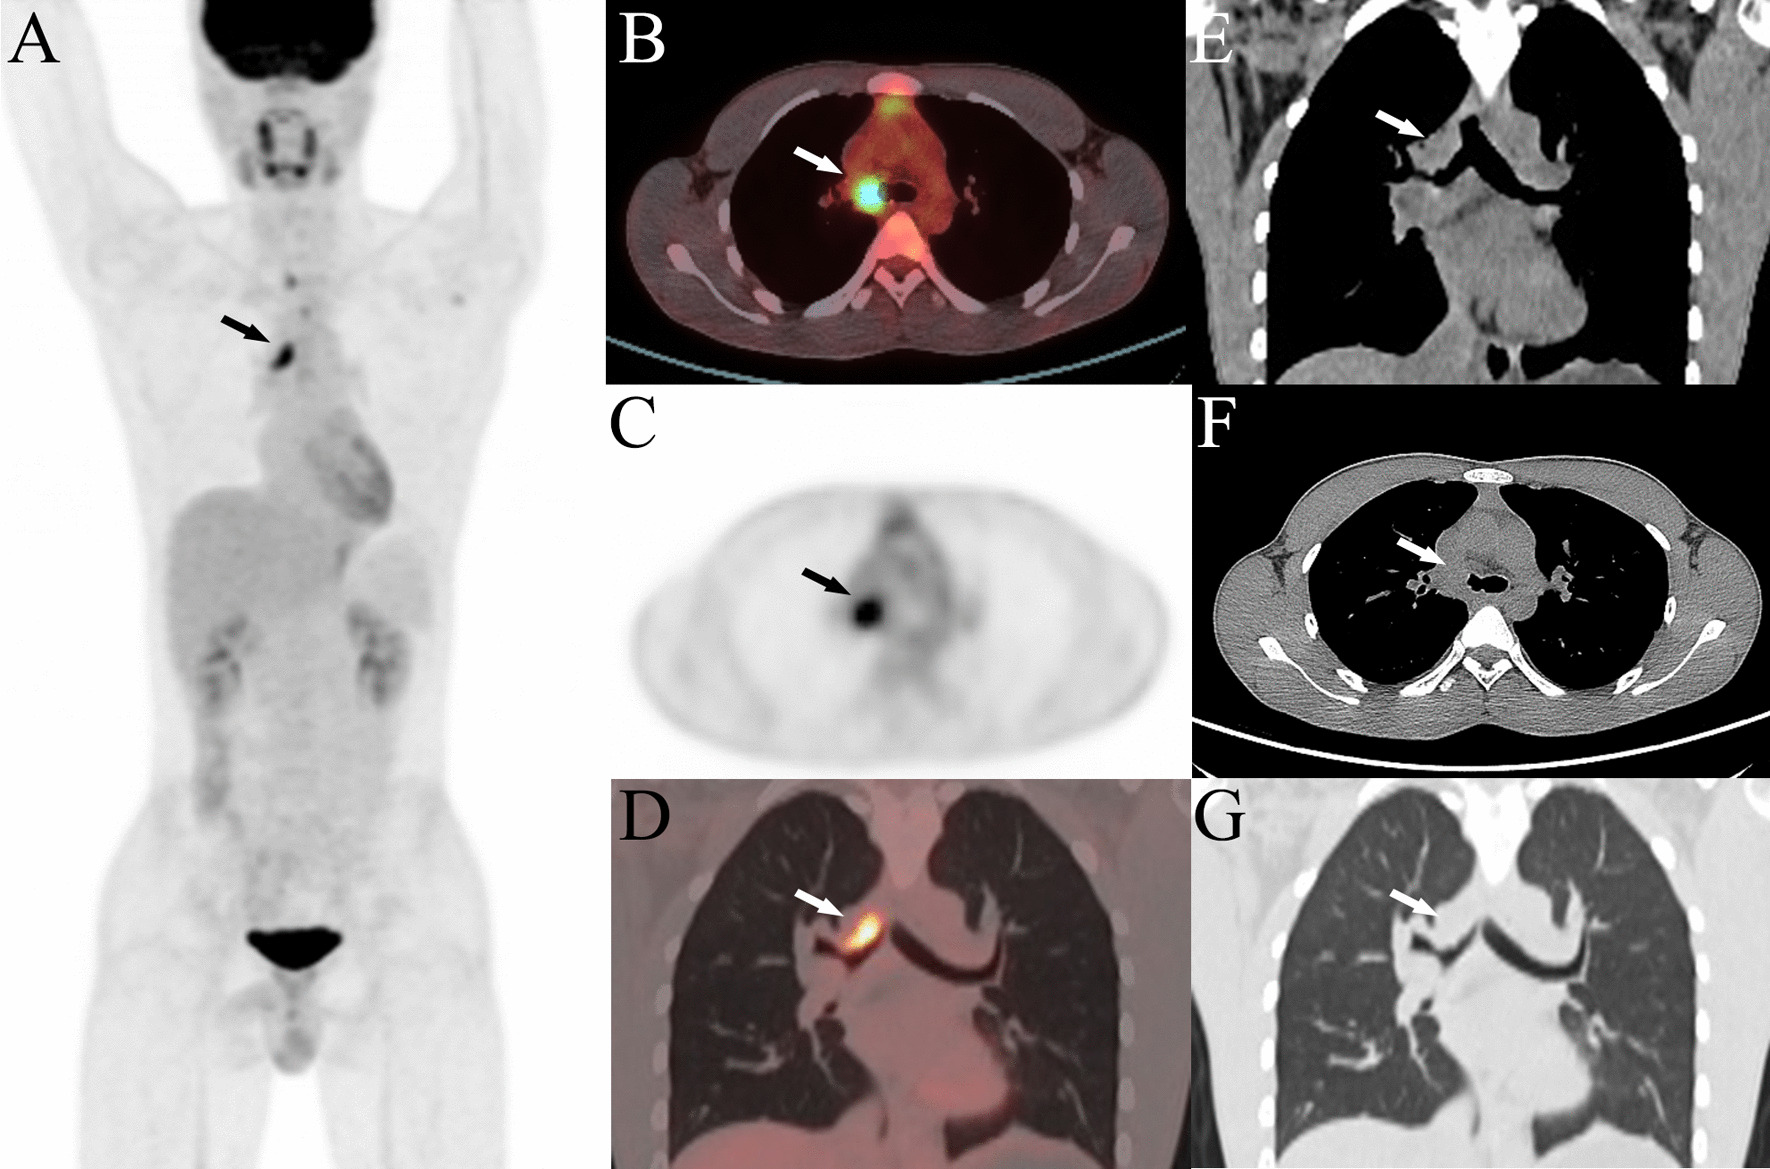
PET/CT image of the RDD patient. Maximum density projection (A), transverse mediastinal window (B), transverse PET image (C) and coronal lung window PET/CT fusion image (D) showed high uptake (arrows) of the right main bronchial wall with SUVmax 8.54 and SUVmean 5.08. Coronal mediastinal window (E), transverse mediastinal window (F) and coronal lung window of CT (G) showed the neoplasm (arrows) in the right main bronchus
